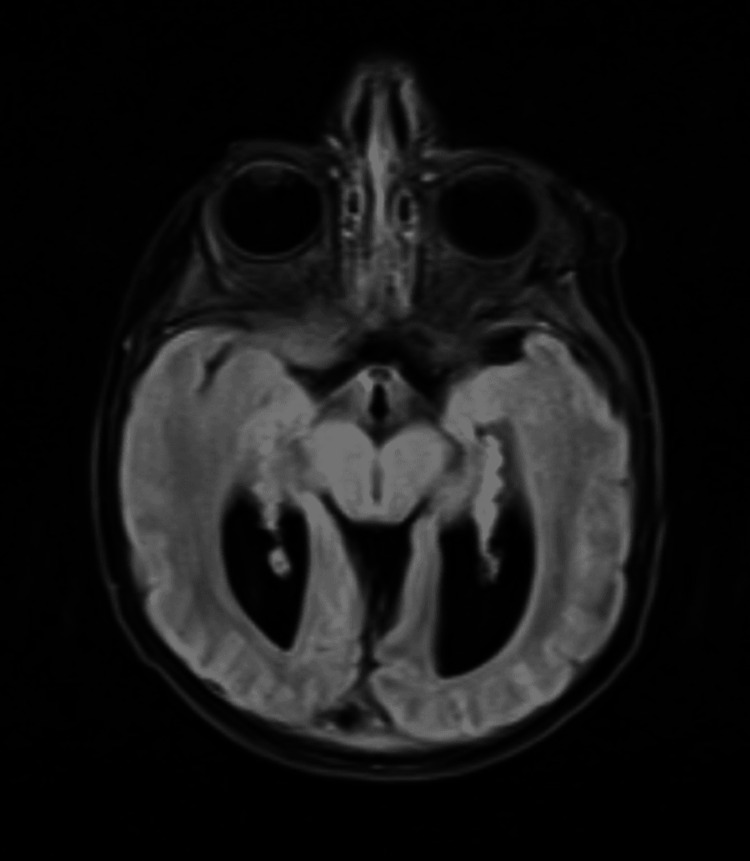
MRI brain (T2 axial) showing colpocephalic configuration of the ventricular system and absence of the corpus callosum (motion artifact present).
